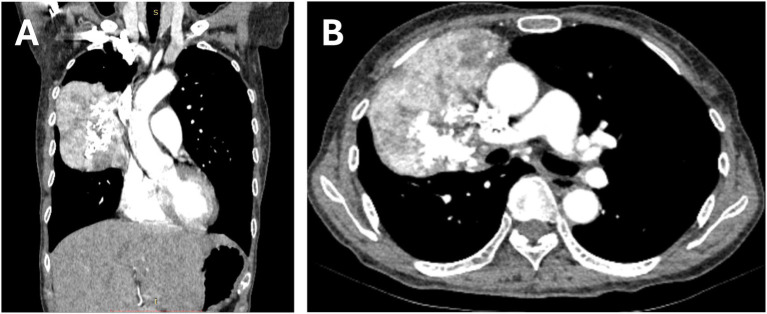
Chest CT imaging. (A) Coronal CT image; (B) cross-sectional CT image of the tumor.
